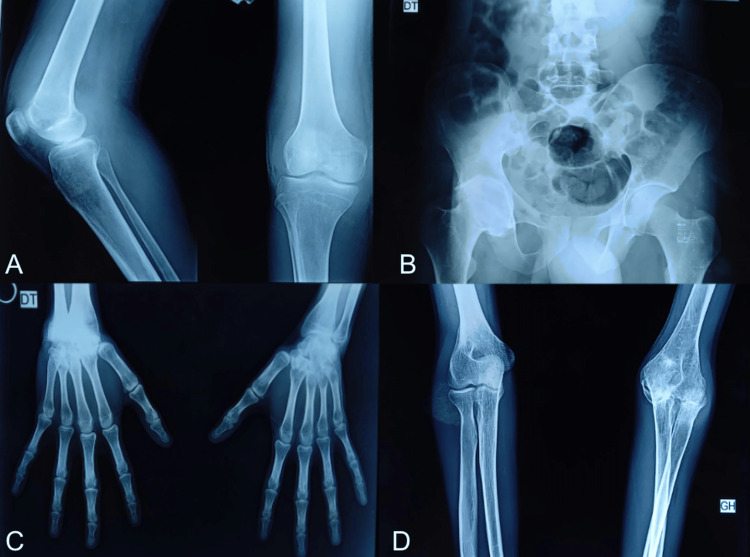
Conventional radiograph findings Demonstrating advanced structural damage of the right knee with joint space narrowing (A), bilateral hip involvement (B), right carpal fusion (C), and fixed flexion deformity of the left elbow (D).
